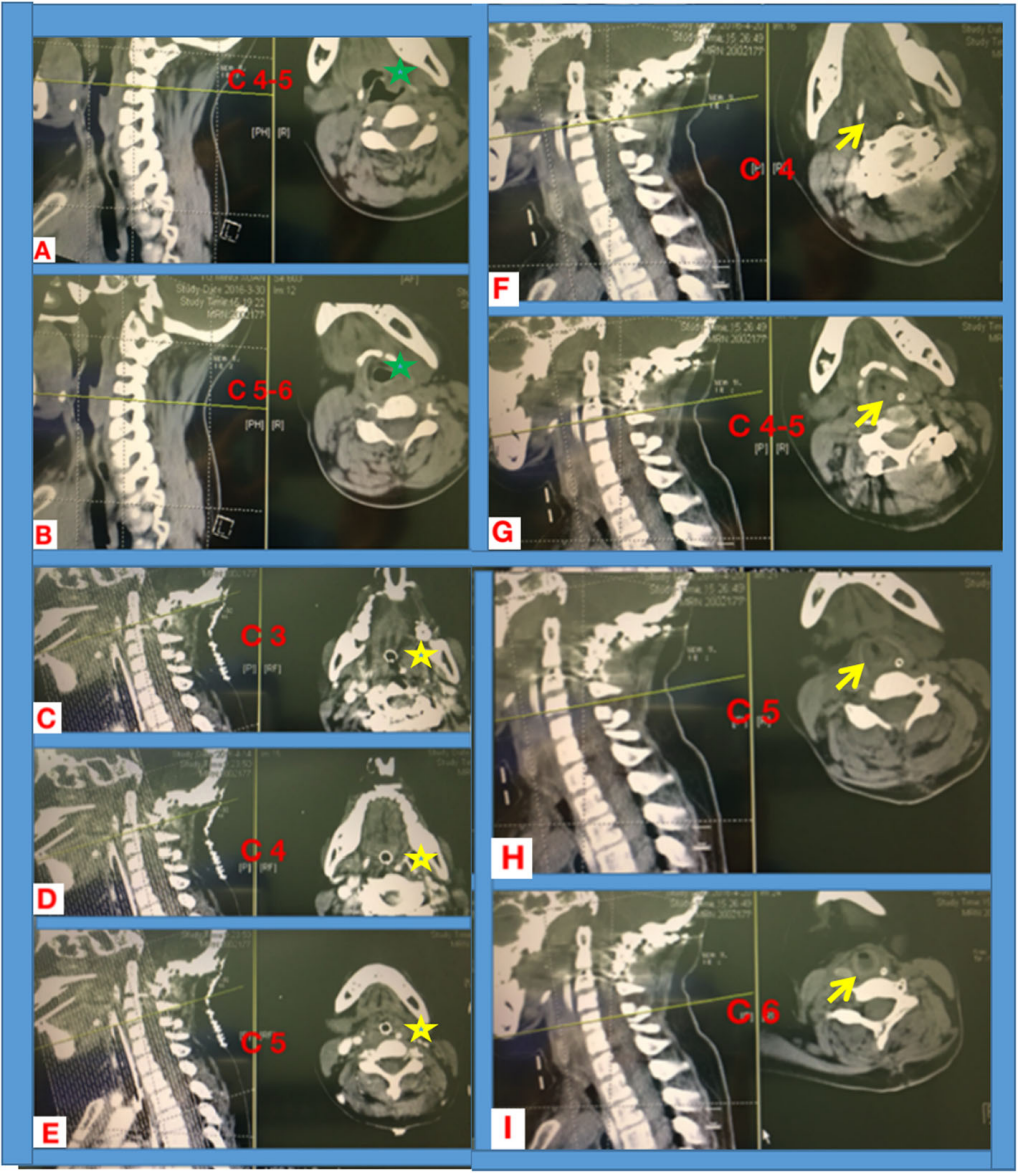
Cervical coronal and corresponding transverse section imaging of CT scanning. Preopeartive imaging showing no obstruction in upper airway in cervical vertebrae 4-6 segement (a, Cervical vertebrae 4-5 and b, Cervical vertebrae 5-6; green asterisk, trachea). On postoperative day 9, no space between tracheal tube and surrounding tissue was noticed from CT scanning images, indicating severe airway edema (c-e, corresponding to Cervical vertebrae segement of 3-5; yellow asterisk, showing swollen Oropharyngeal cavity). On postoperative day 15 (5 days after trecheostomy), airway stenosis still existed with significant edema, corresponding to the level of Cervical vertebrae segement of 4-6, (f-i; corresponding to C4-6 segement; yellow arrow, airway edema and resultant tracheal stenosis)
